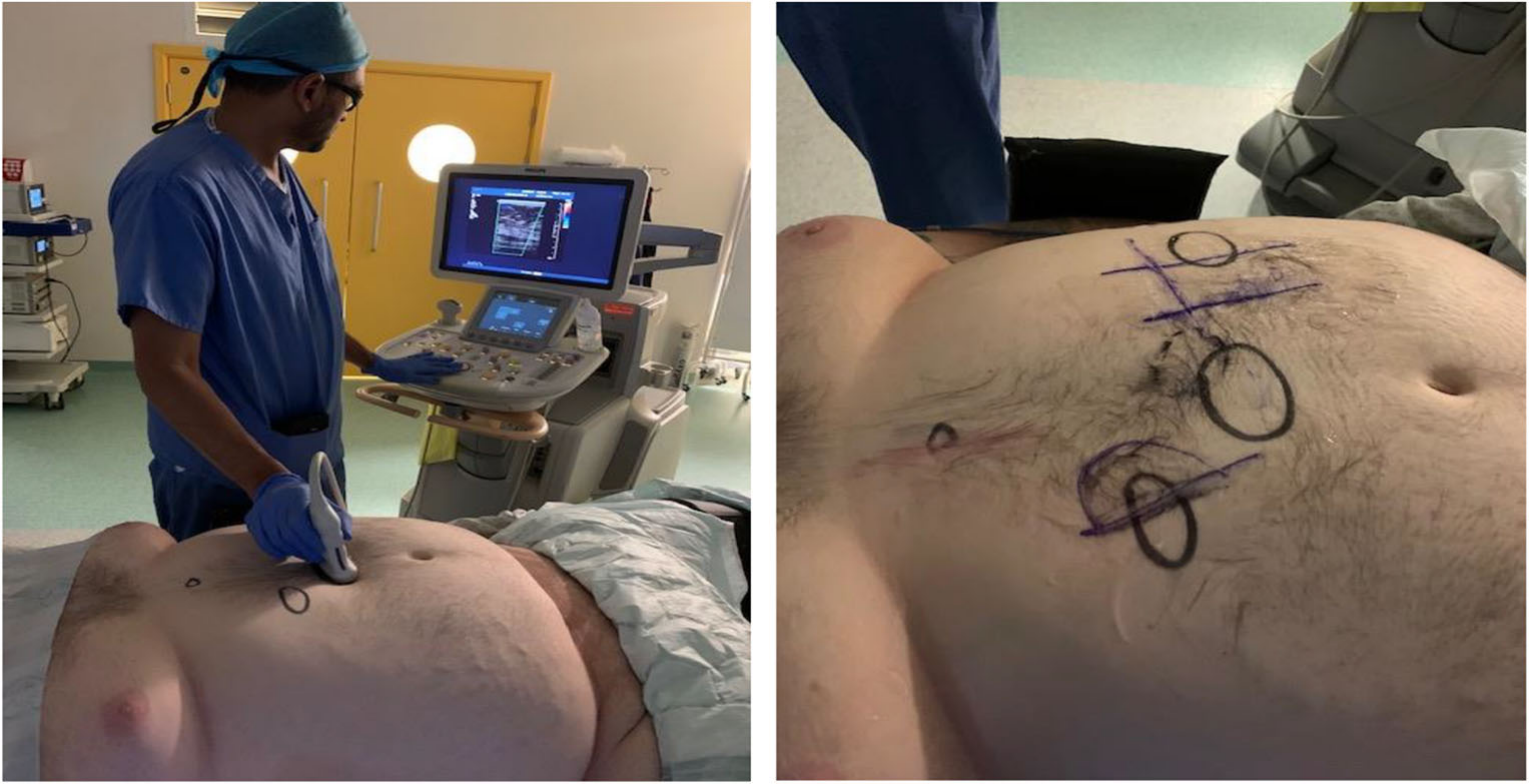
Pre-op ultrasound-guided marking of port sites prior to laparoscopic sleeve gastrectomy
